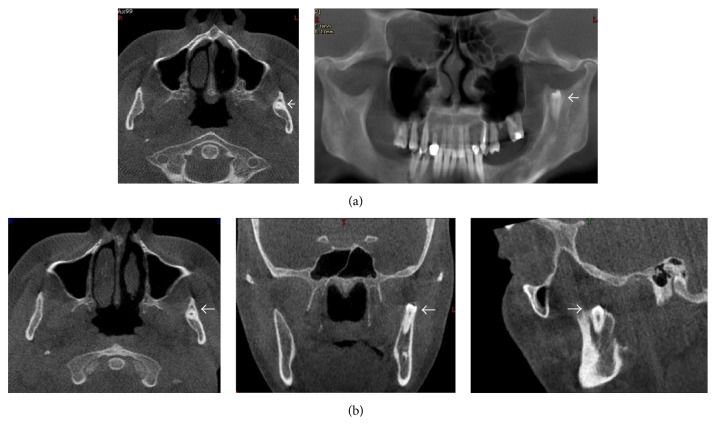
(a, b) CBCT scans show that the premolar is located in the sigmoid notch. (a) Panoramic radiography reconstructed by CBCT and (b) MPR-CBCT: (I) axial slice, (II) coronal slice, and (III) sagittal slice.
